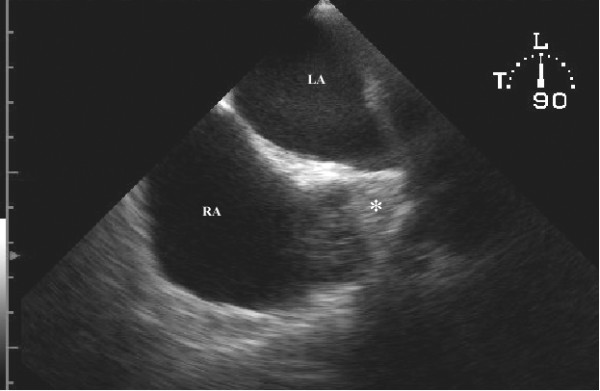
Transesophageal echocardiography in right atrial long axis two-dimensional echocardiographic view demonstrates absence of right vena cava superior (black star) (LA = left atrium, RA= right atrium).
